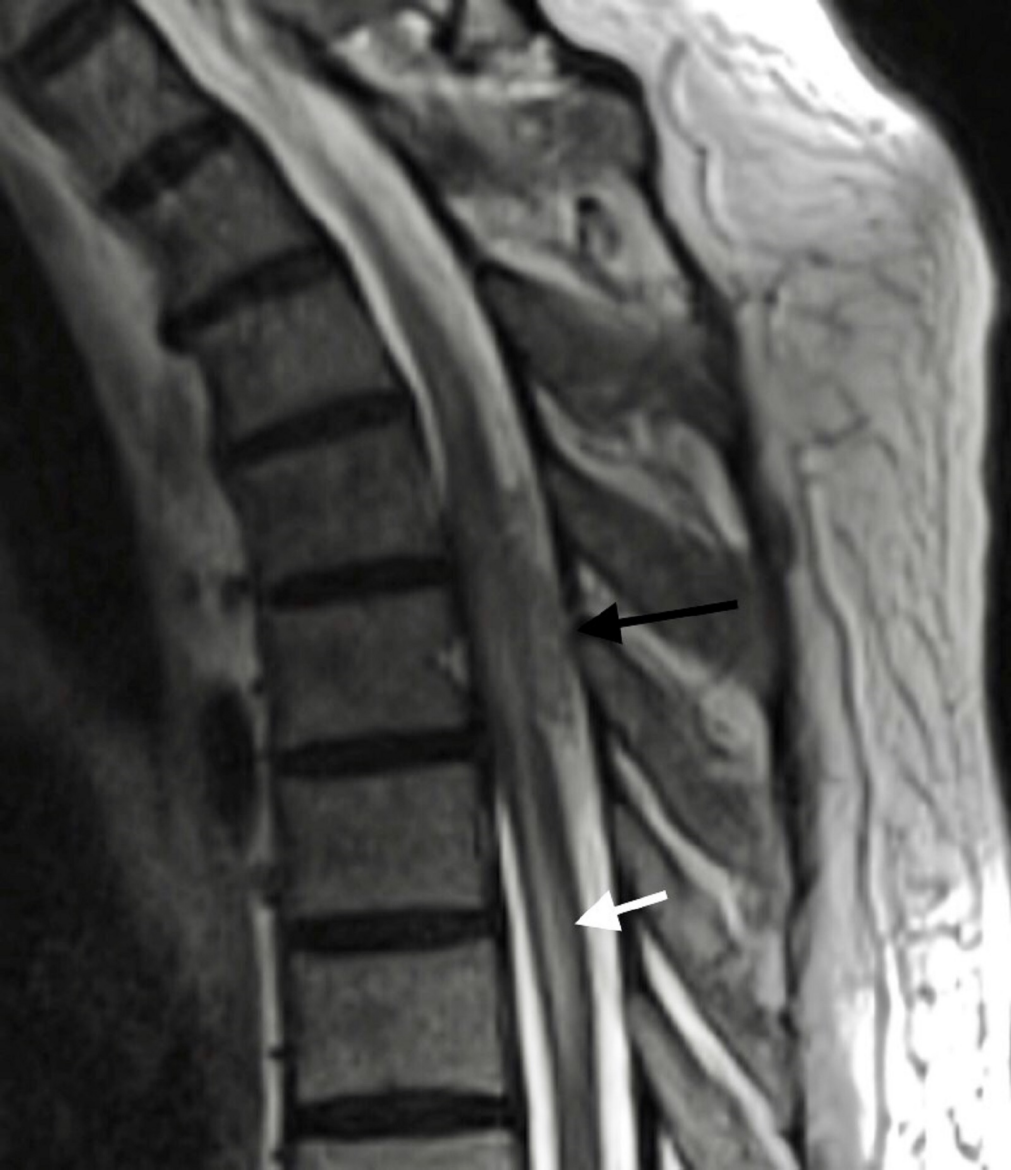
Thoracic T2-weighted magnetic resonance imaging (MRI). Sagittal T2-weighted MRI of thoracic spine at the level of T5 and T6 demonstrating an extraaxial appearing collection (black arrows) and spinal cord edema (white arrow).
